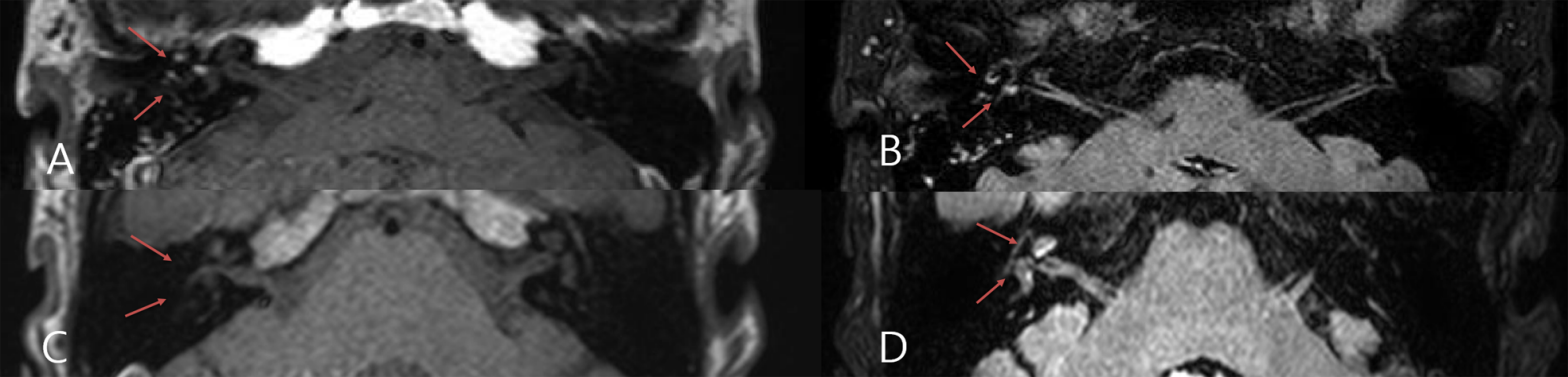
MRI findings of intra-labyinthine hemorrhage (A,B) and labyrinthis (C,D). Labyrinthitis and intra-labyrinthine hemorrhage (ILH) both show a high signal intensity on a 3D fluid-attenuated inversion recovery sequence (B,D), but the high signal intensity on the precontrast T1 sequence suggests labyrinthine hemorrhage (A) rather than labyrinthitis (C).
